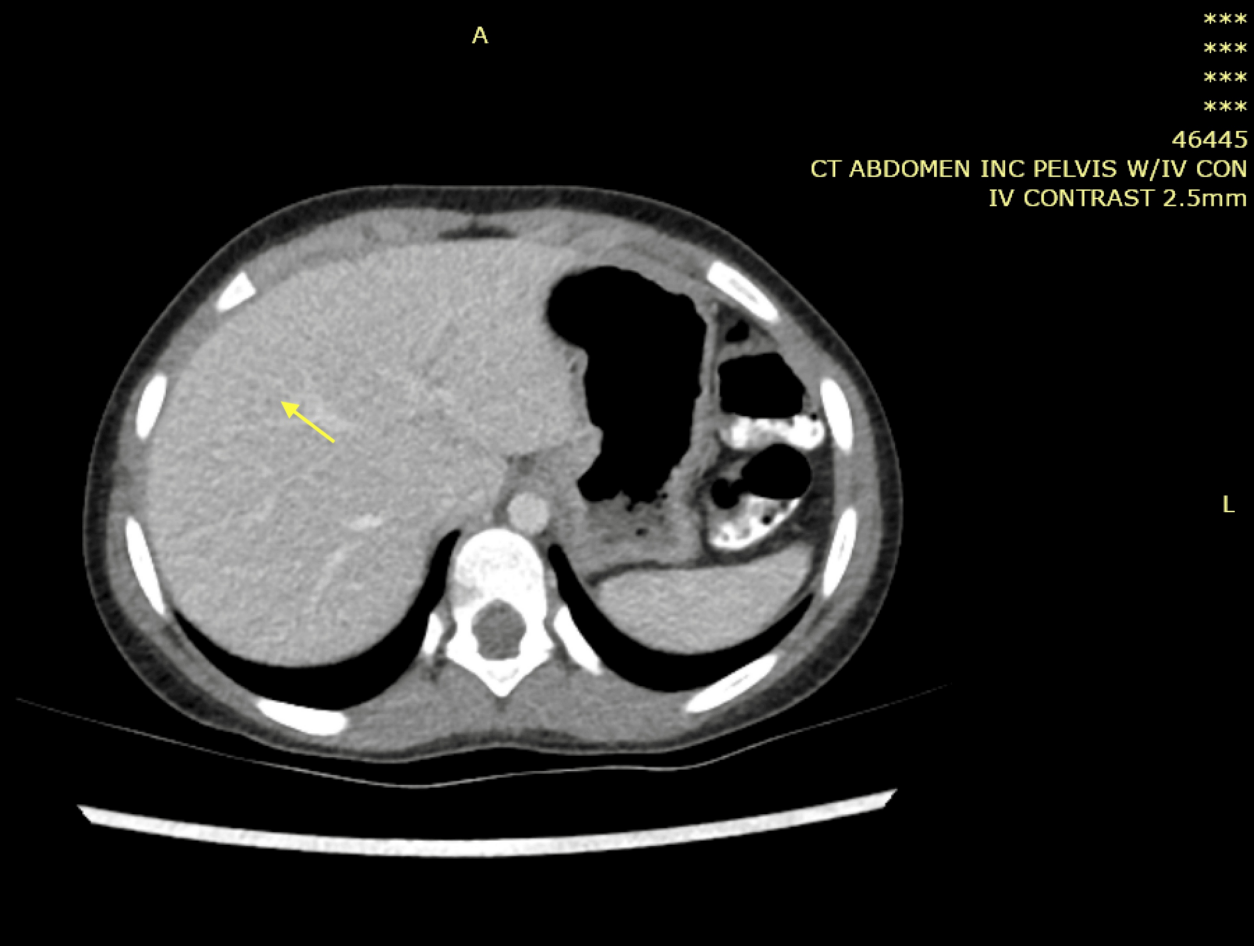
Computed tomography of the abdomen with IV contrast showing complete resolution of the two previously seen hepatic lesions (yellow arrow).
